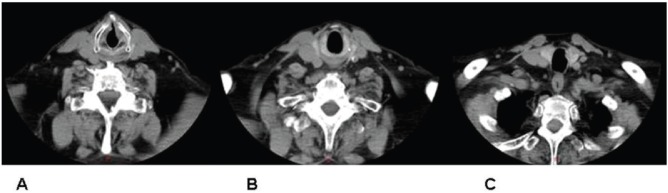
CT indicating both glottic (A) and tracheal (C) tumours. Intermediate tissue appears grossly normal (B).
